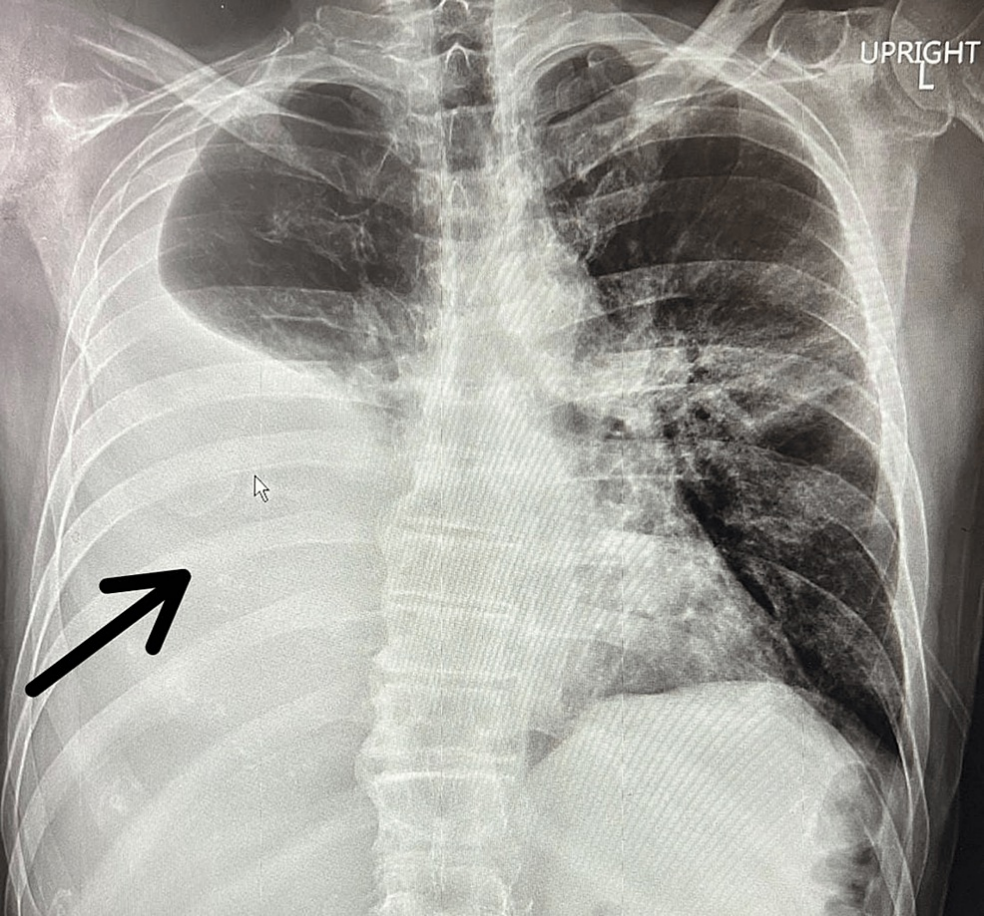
Chest X-ray showing a large right-sided pleural effusion.
